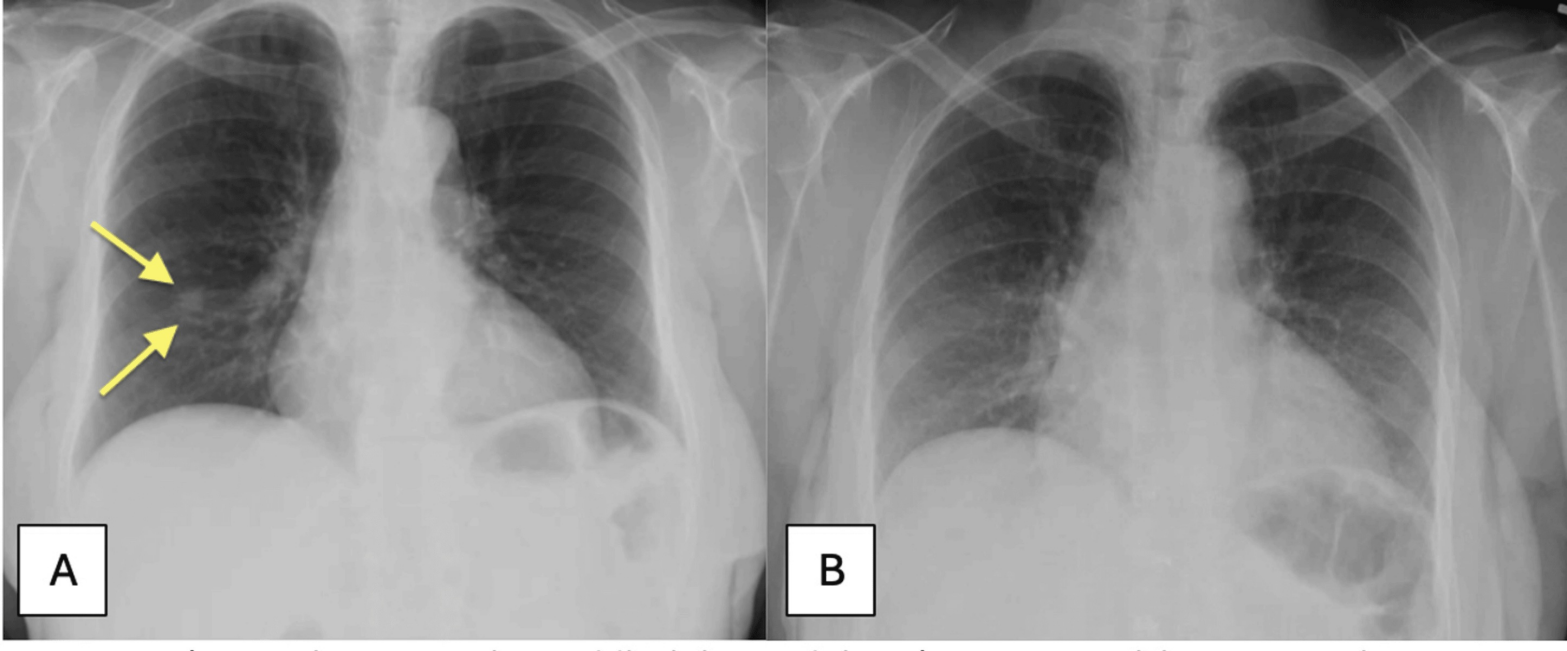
(A) CXR showing a nodule in the right middle lobe. (B) CXR normal lung parenchyma one year prior. CXR, chest X-ray
